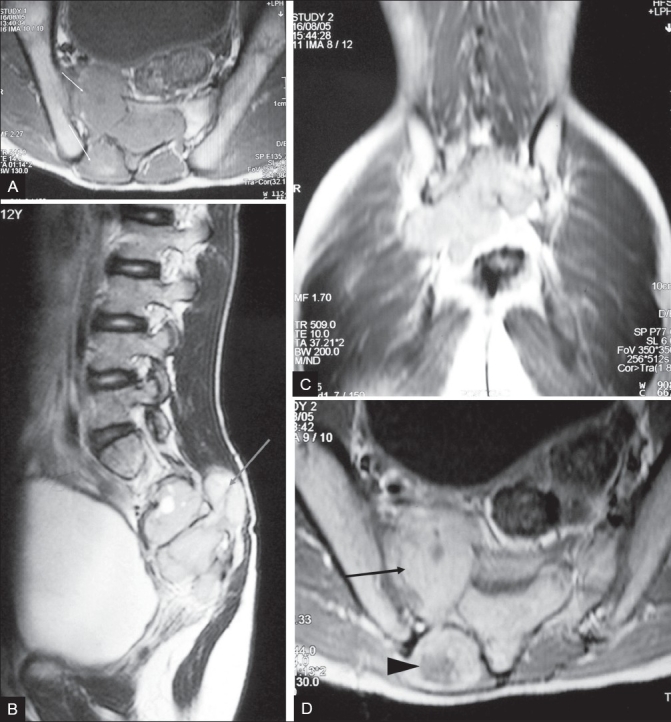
T1W axial (A) and T2W mid-sagittal (B) MRI images of the LS spine show a lobulated soft tissue mass (arrows), isointense to pelvic muscles on T1W and hyperintense on T2W images with areas of necrosis; both intraspinal and extraspinal components cause widening of the sacral spinal canal and sacral foramina. T2W coronal image (C) shows clubbed, finger-like extensions along the path of the sacral nerves. Axial T1W contrast-enhanced image (D) shows intense enhancement with areas of low intensity within, corresponding to necrotic areas. Involvement of the pyriformis (arrow) and right erector spinae muscles (arrowhead) can be seen
